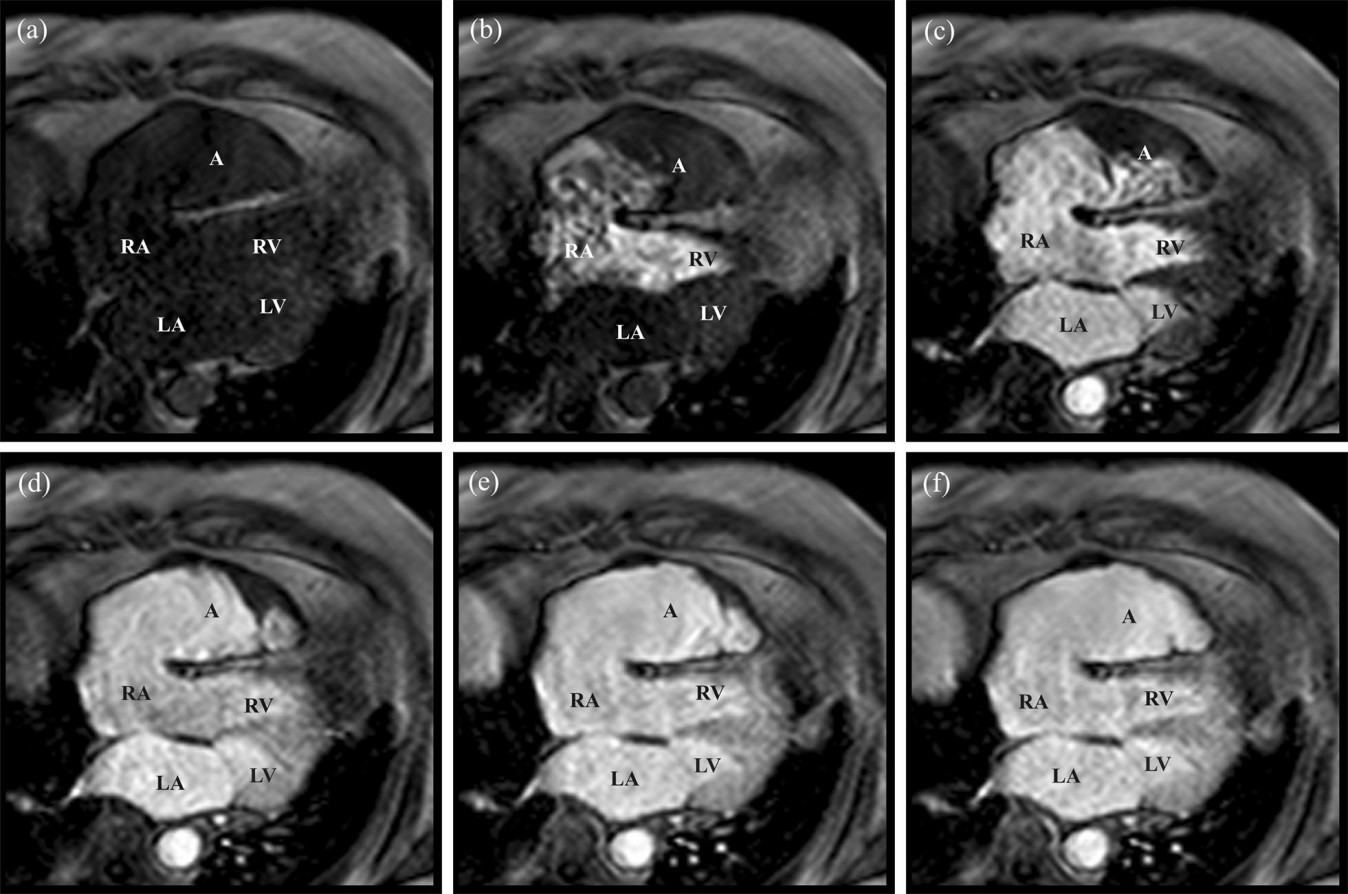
Axial static images of the first pass perfusion cine taken with administration intravenous gadolinium. Prior to injection of contrast material, a the right atrial appendage aneurysm can be visualized compressing the right ventricle. After administration of 25 mL gadolinium IV contrast agent (Multihance^®^), there is sequential opacification of the right (b) and the left (c) cardiac chambers. Afterwards, there is slow, progressive contrast opacification of the stagnant blood pool in the right atrial appendage aneurysm until complete contrast opacification of the aneurysm (d–f). This indicates that the original area of hypoenhancement found on computed tomography was in fact due to slow flow, not a thrombus. A, RAA aneurysm; LA, left atrium; LV, left ventricle; RA, right atrium; RV, right ventricle
